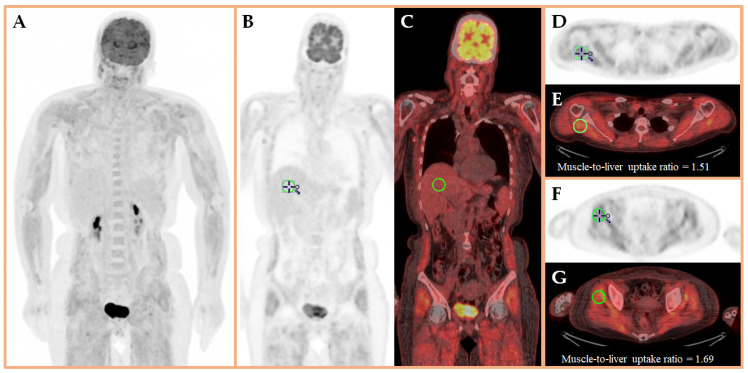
A 53-year-old woman with triple-negative breast cancer (TNBC), classified as cT2N1M0 [1], received neoadjuvant therapy with pembrolizumab and cytotoxic chemotherapeutic agents [2]. After the first cycle, she developed generalized pain, weakness, rash, and edema over the trunk and limbs, eventually becoming bedridden. Fluorine-18 fluorodeoxyglucose positron emission tomography (^18^F-FDG PET) was performed to rule out disease progression and skeletal metastasis. The patient fasted for at least 4 h before intravenous ^18^F-FDG injection (400.00 MBq). The patient did not exercise before the injection. We performed the ^18^F-FDG PET scan from head to thigh 60 min after the radiotracer injection. (A): Maximum Intensity Projection showing diffusely increased uptake in the muscles without skeletal or visceral metastasis. Coronal (B,C) and axial PET and fused PET/CT images showed the scapular (D,E) and gluteal (F,G) muscle-to-liver mean standardized uptake value (SUV_mean_) ratios of 1.51 and 1.69, respectively. Laboratory tests showed elevated creatine kinase at 1945 U/L (reference range: 30–223 U/L) [3] and positive anti-transcription intermediary factor 1-γ autoantibodies. Taken together, these findings confirmed the diagnosis of paraneoplastic and pembrolizumab-induced myositis [4,5,6].
